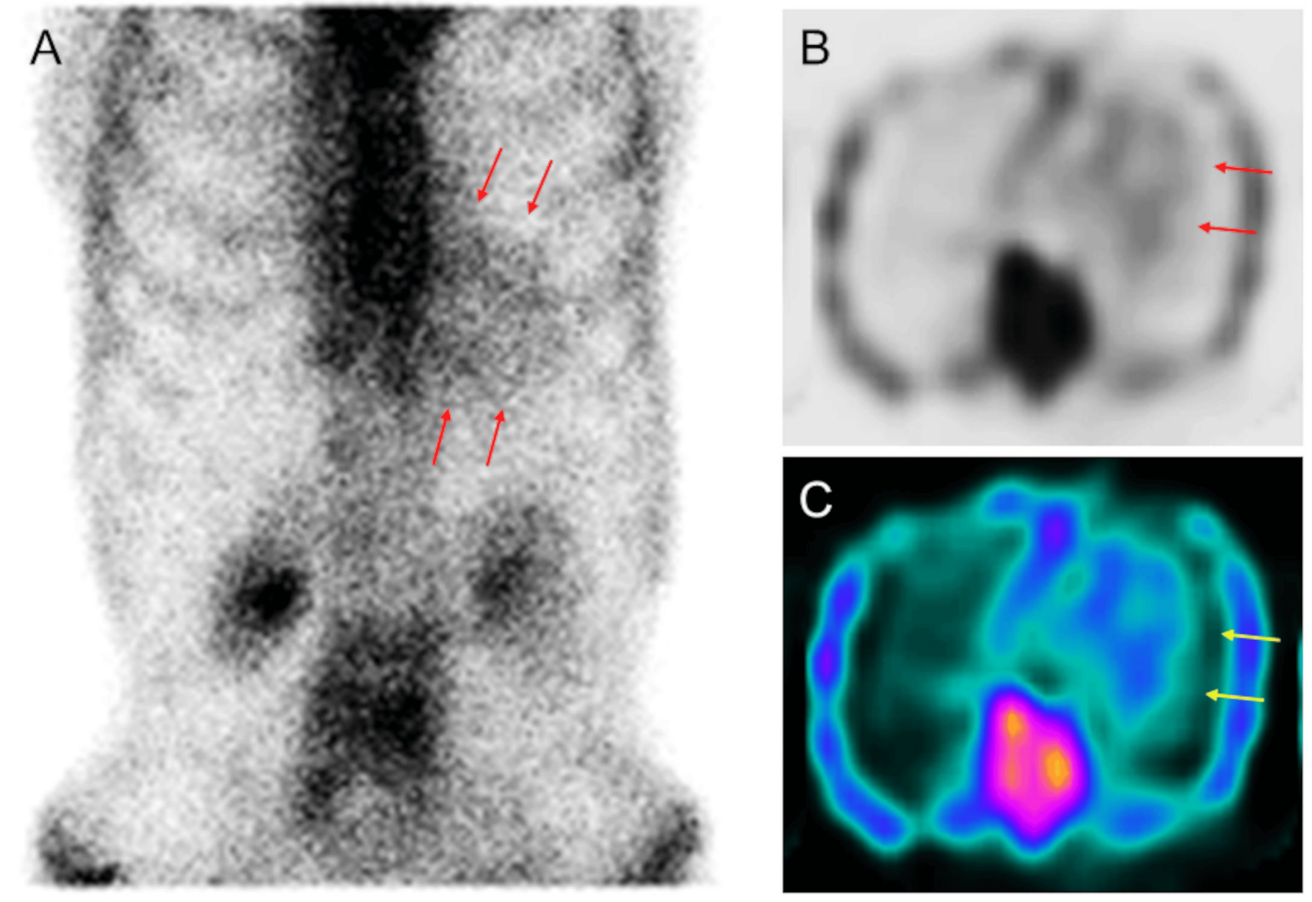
99mTc-PYP scintigraphy 99mTc-PYP scintigraphy demonstrated Perugini grade 2 myocardial uptake (moderate uptake equal to rib uptake), with an increased heart-to-contralateral ratio of 1.40, on both planar (A, arrows) and single photon emission tomographic images (B, C, arrows) at the three-hour mark.
